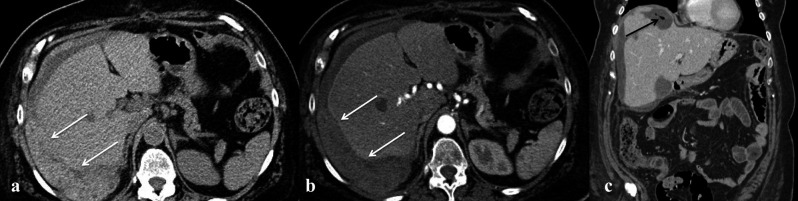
Delayed post contrast CT images oriented on axial (a), coronal (b) and sagittal (c) planes, showing hydroureteronephrosis
